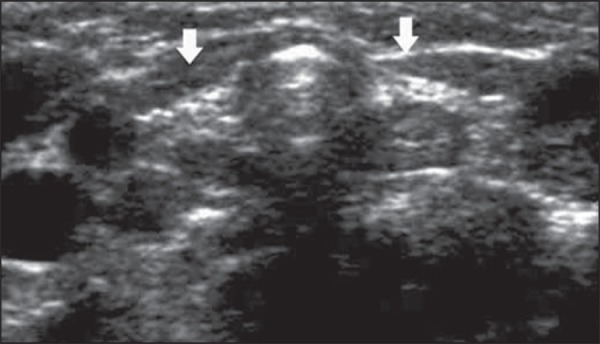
Ultrasonography of cervical region. In this case, the thyroid gland (arrows
